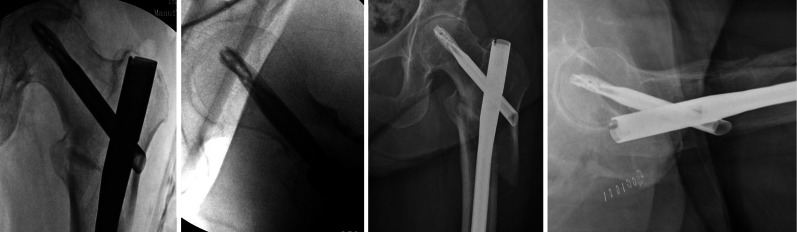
Pictured left are the AP and lateral views of the hip obtained following the index procedure. Pictured right are the repeat radiographs taken 2 weeks postoperatively that show interval displacement of the fracture, loss of reduction, and shortening of the proximal femur
